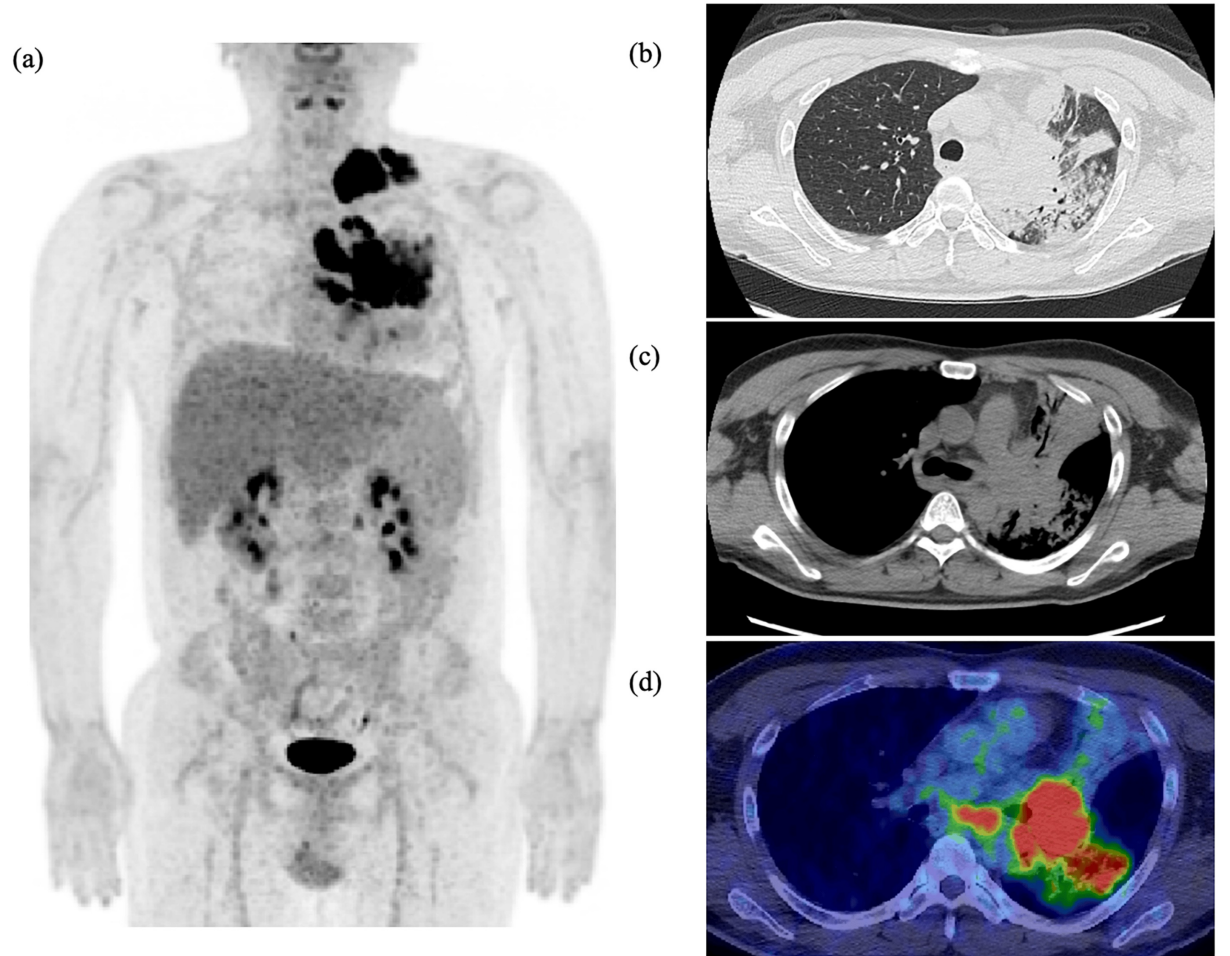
Positron emission tomography-computed tomography (PET-CT) findings of the case. (a) PET-CT on day 208. (b, c) There is a large infiltrative shadow extending from the left upper lobe to the lower lobe. This area shows high uptake with an SUVmax of 17. (d) Additionally, multiple lymphadenopathies are observed at the left hilum, left paratracheal region, left supraclavicular, and left infraclavicular areas, all demonstrating high uptake.
